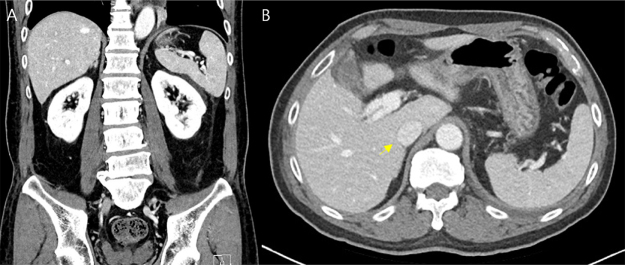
The CT scan taken on the 5th day of hospitalization. (a) Coronal; (b) axial cut. The CT showed that almost all of the fluid was drained from the abdominal cavity. In the coronal cut, the size of the internal organs had recovered, and the level of both diaphragms had descended to the normal level. It was a remarkable finding that the IVC had fully recovered its round shape from the narrowed shape in the axial cut (yellow arrow).
